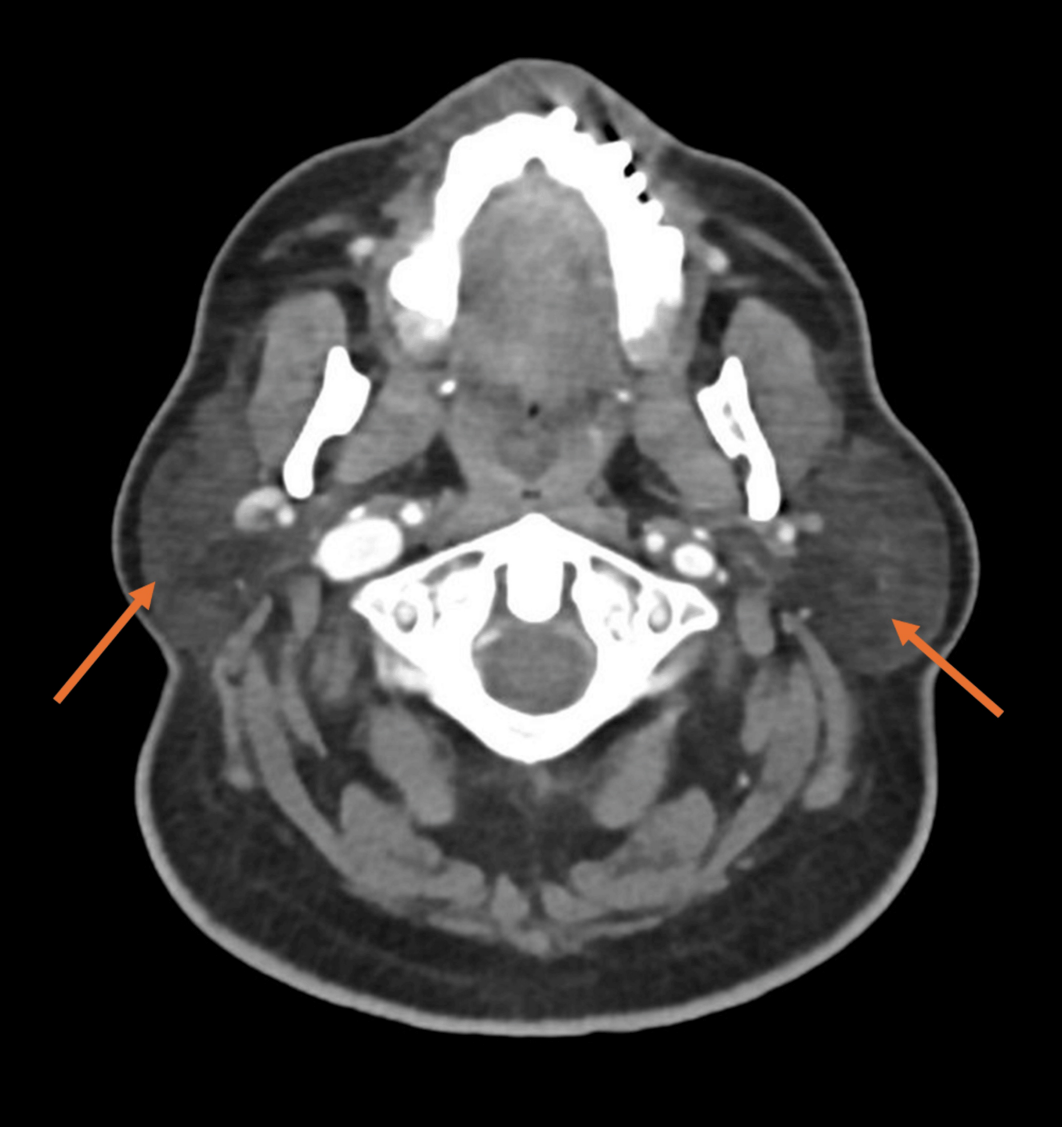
Coronal CT scan of the head shows bilateral parotid gland enlargement.
